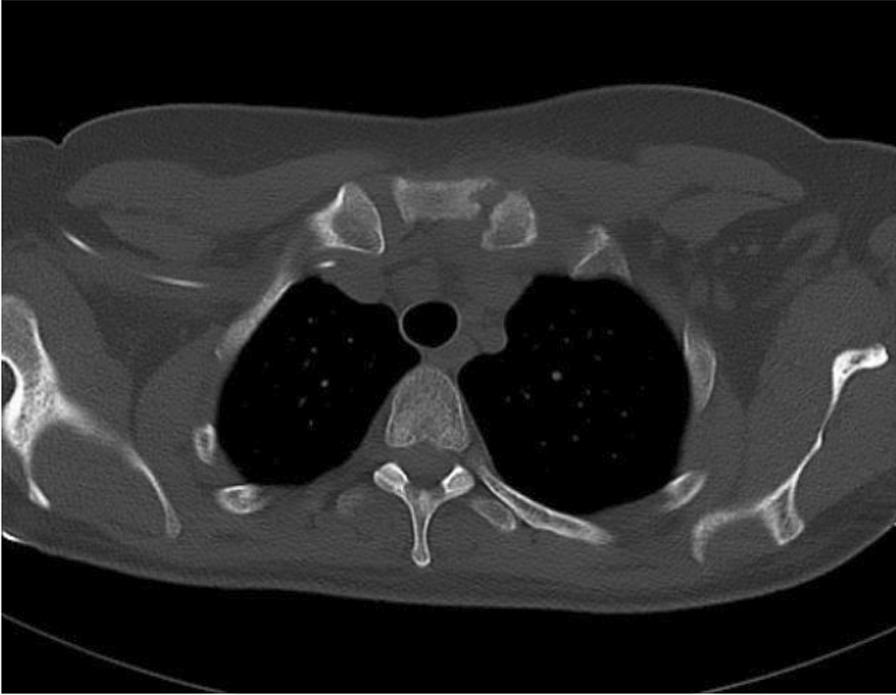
Repeat computed tomography showed increased erosions of the left SC joint
